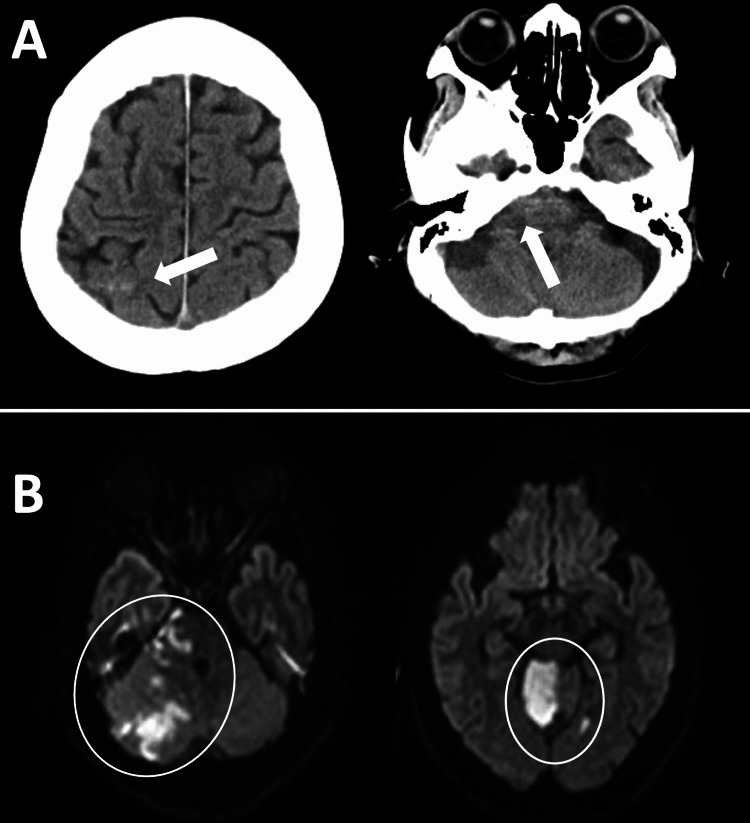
Neurodiagnostic images A) The non-enhanced computed tomography image on admission showed a high-density area (HDA) considered to be subarachnoid hemorrhage (arrows). The HDA of the left image was confirmed as subarachnoid hemorrhage. However, the HDA of the right image was confirmed as an intracranial abscess lately. B) Magnetic resonance imaging diffusion-weighted image of cerebellar infarction on admission (circled).
